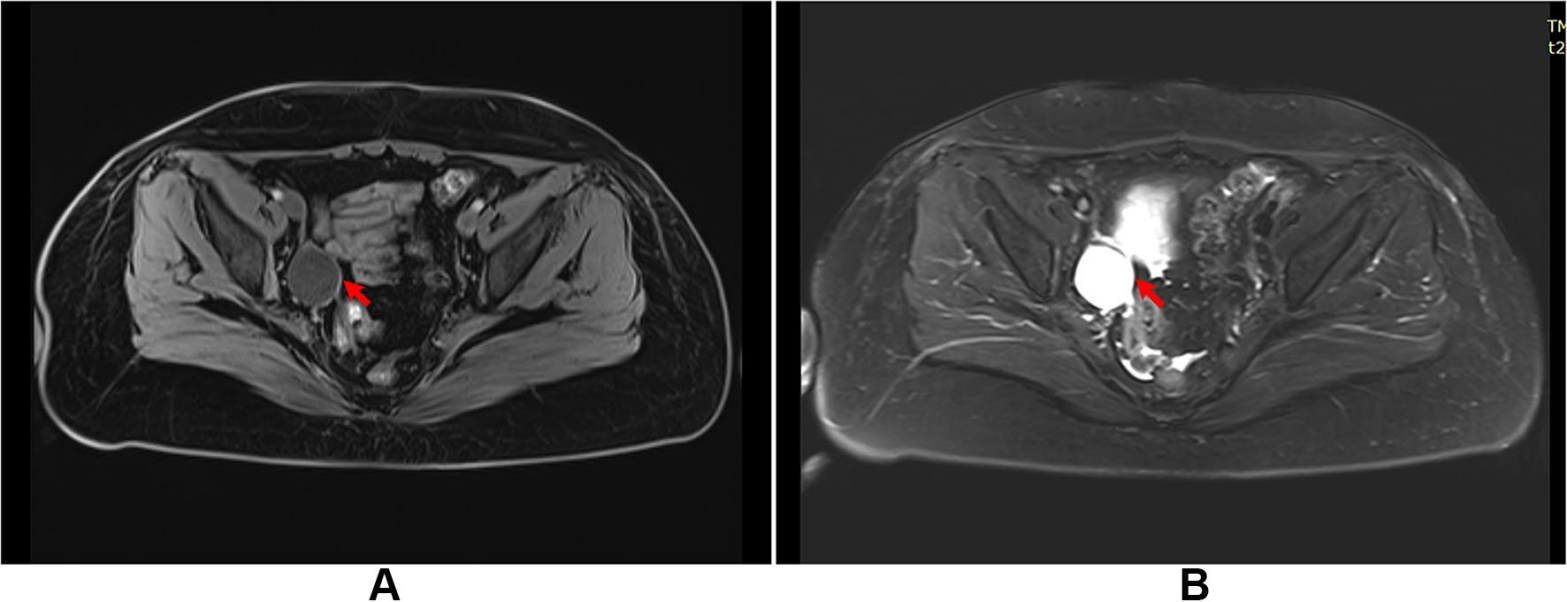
An infected lymphocele scanned by MRI showing hypo-intense T1-weighted (a) and hyper-intense signals on T2-weighted images (b)
